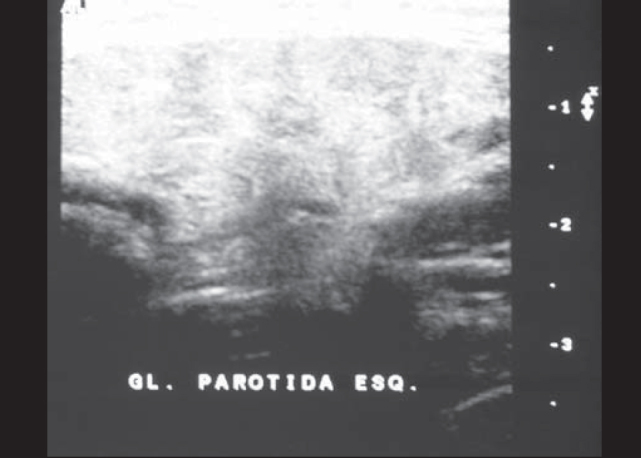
FDO Ultrasound (Case 1): Six years of progression, normal pattern.
